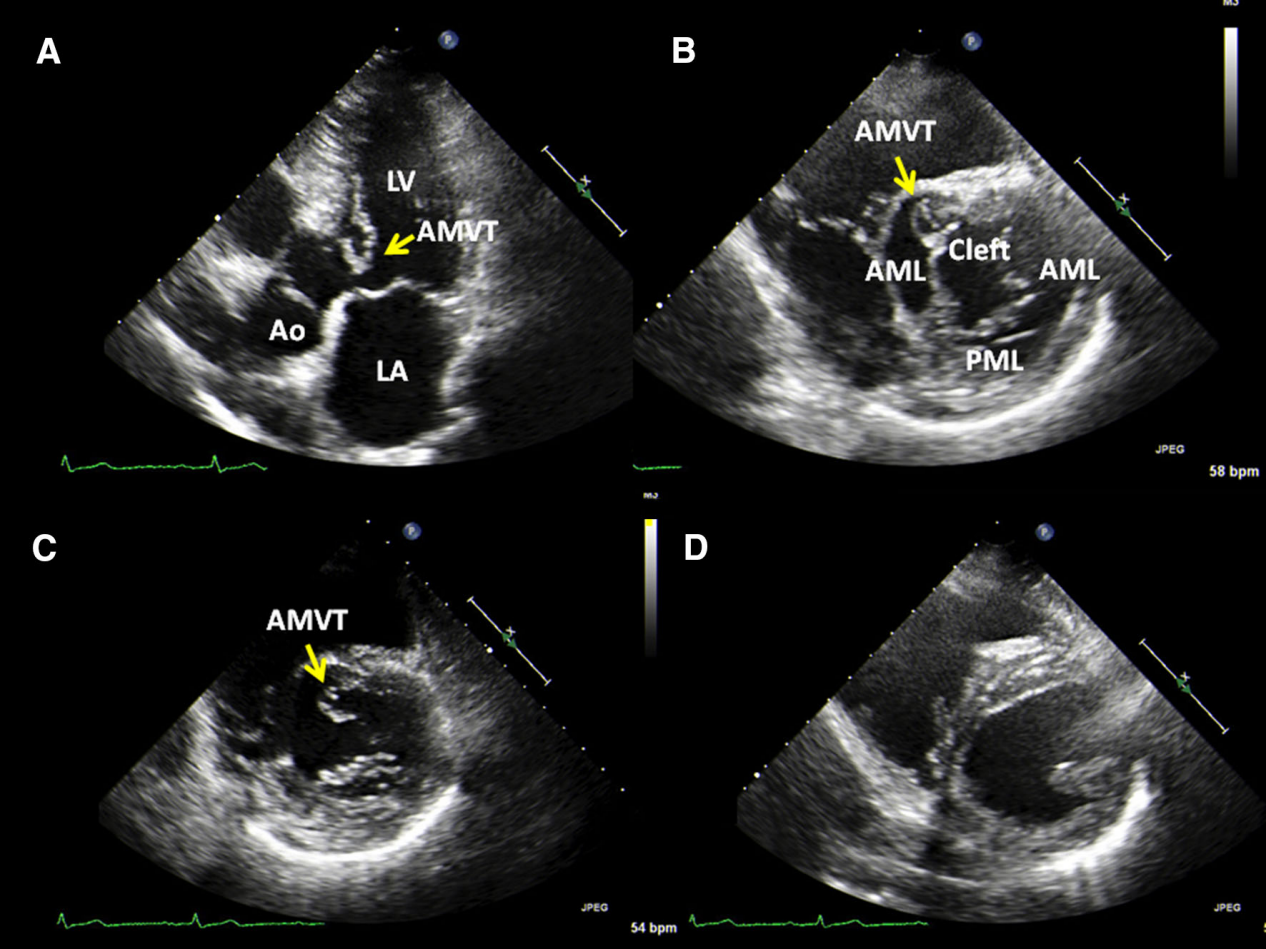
a Transthoracic echocardiography in the apical long-axis view shows accessory mitral valve tissue (AMVT) (*arrow*) into the left ventricular outflow tract (LVOT). *LV* left ventricle, *LA* left atrium, *Ao* aorta, *AMVT* accessory mitral valve tissue. b Transthoracic echocardiography in the parasternal short-axis view in diastole shows mitral valve leaflet cleft and AMVT (*arrow*) attached from the base of the intraventricular septum to anterior mitral leaflet. *AML* anterior mitral leaflet, *PML* posterior mitral leaflet. c Transthoracic echocardiography in the parasternal short-axis view in systole. d Transthoracic echocardiography in the parasternal short-axis view shows single papillary muscle (loss of posterior papillary muscle)
